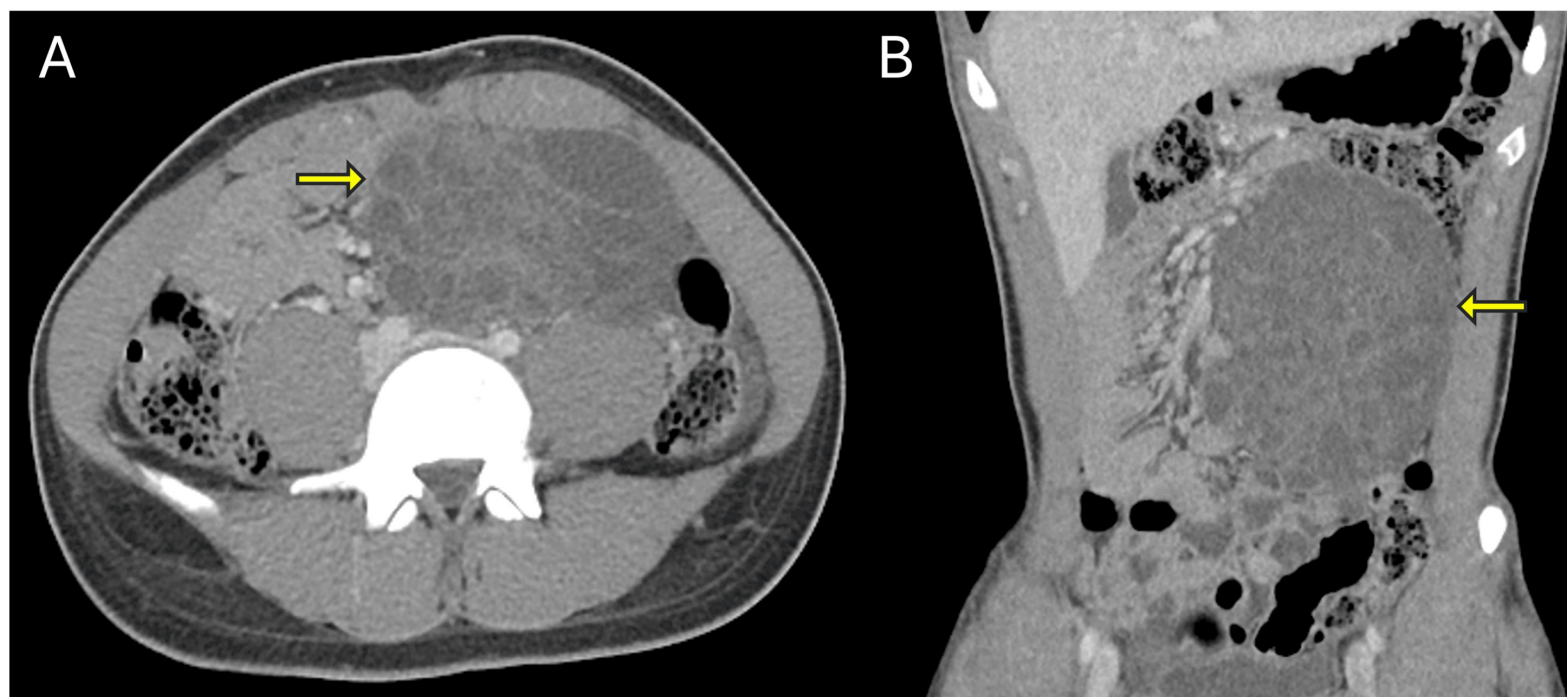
Axial (A) and coronal (B) contrast-enhanced CT images of the abdomen demonstrating a large, well-defined, multiloculated cystic mass (yellow arrows) occupying the left retroperitoneal space. The lesion displays low attenuation with internal septations and mass effect on adjacent bowel loops without evidence of obstruction. CT: computed tomography
